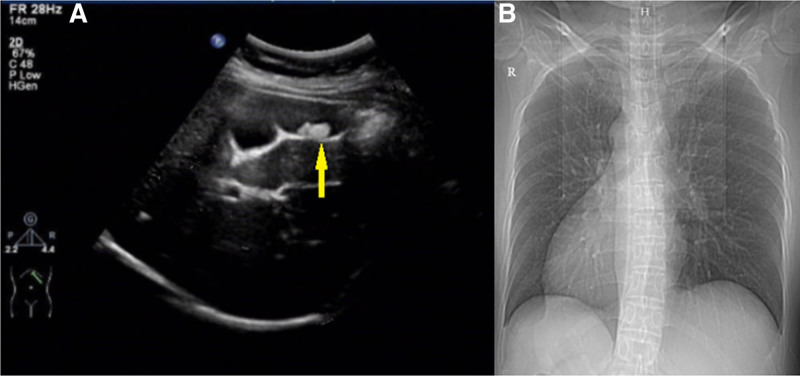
(A) Abdominal ultrasound image of the left upper quadrant showing a gallbladder with multiple stones (yellow arrow) (B) Chest X-ray demonstrated dextrocardia.
